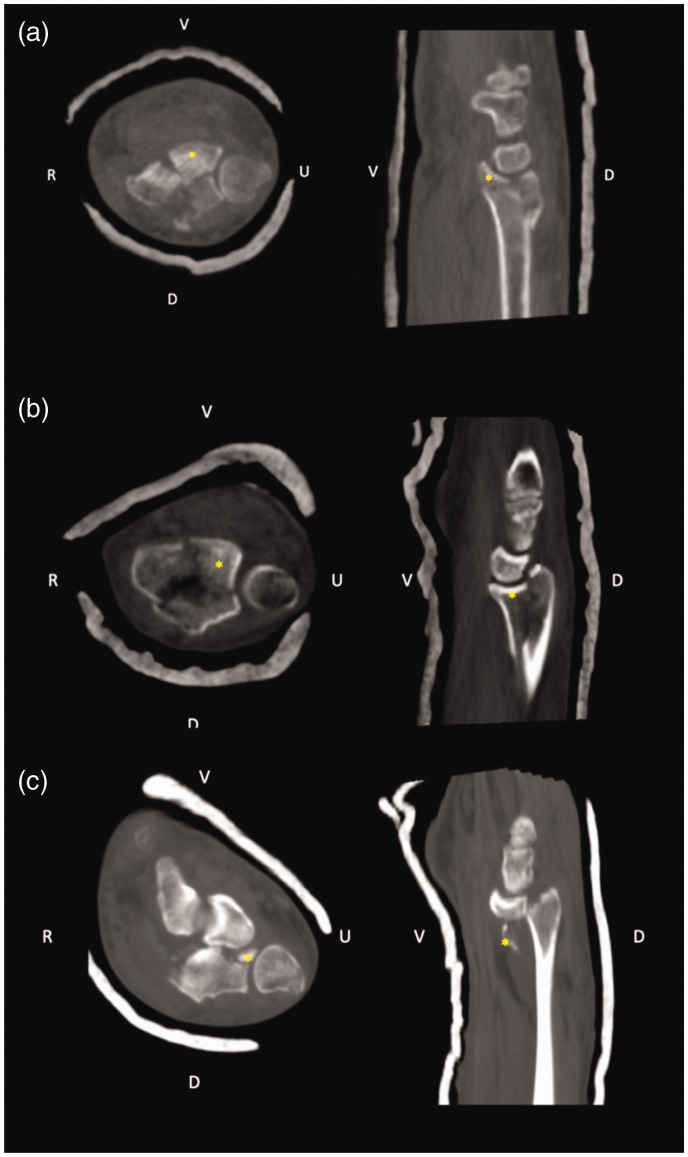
Computed tomography reconstructions demonstrating the axial and sagittal profiles of the (a) type 1 dorsally extended fragment, (b) type 2 large palmar shear fragment and (c) type 3 small palmar rim fragment. Asterisk denotes the palmar ulnar corner fragment.
